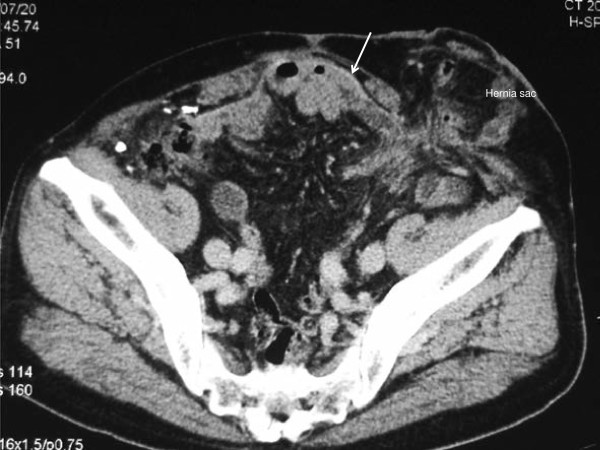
Preoperative computed tomography image of case 2. The redundant sigmoid colon is protruding from the stoma's lateral aspect into the hernia sac. A prosthetic mesh (arrow) previously placed for the midline incisional hernia is visualized.
